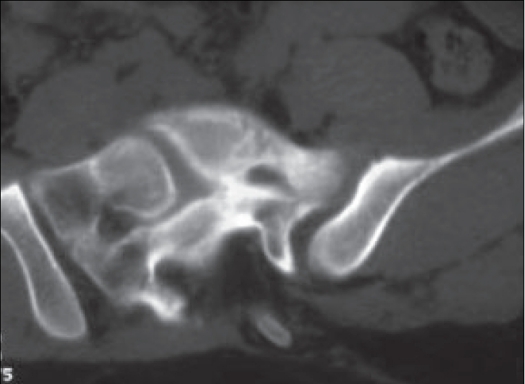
MRI showing the bony strut with spinal dysraphism, lipomyelomeningocele and dermal sinus
